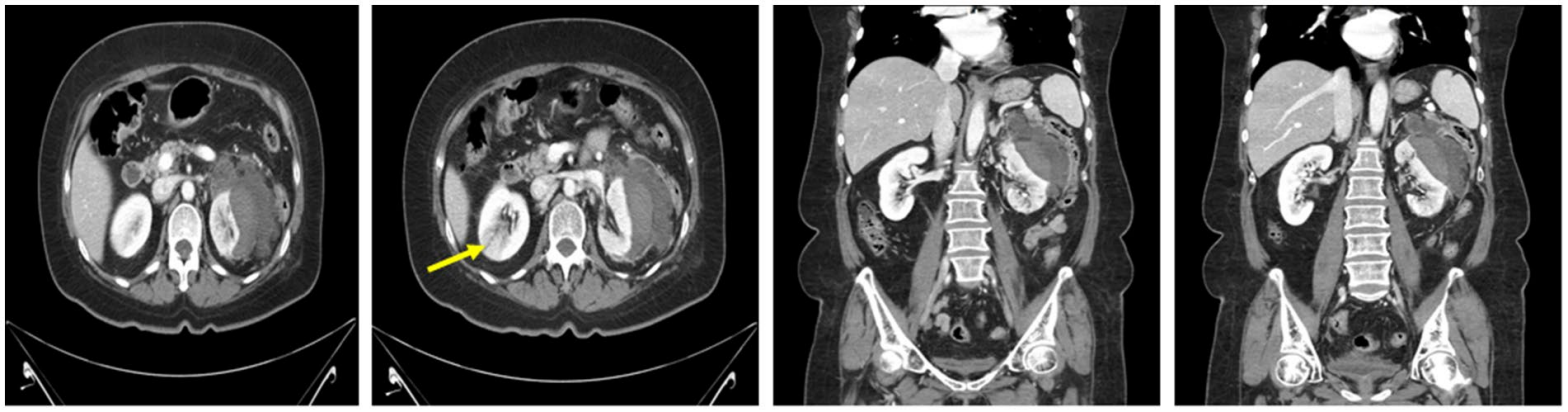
Contrast-enhanced CT showing a non-enhancing subcapsular hematoma measuring 8 cm in length on the left kidney, accompanied by fluid collection in the perinephric space and suspicious wedge-shaped heterogeneous areas of poor enhancement (arrow) representing acute pyelonephritis in the right kidney.
